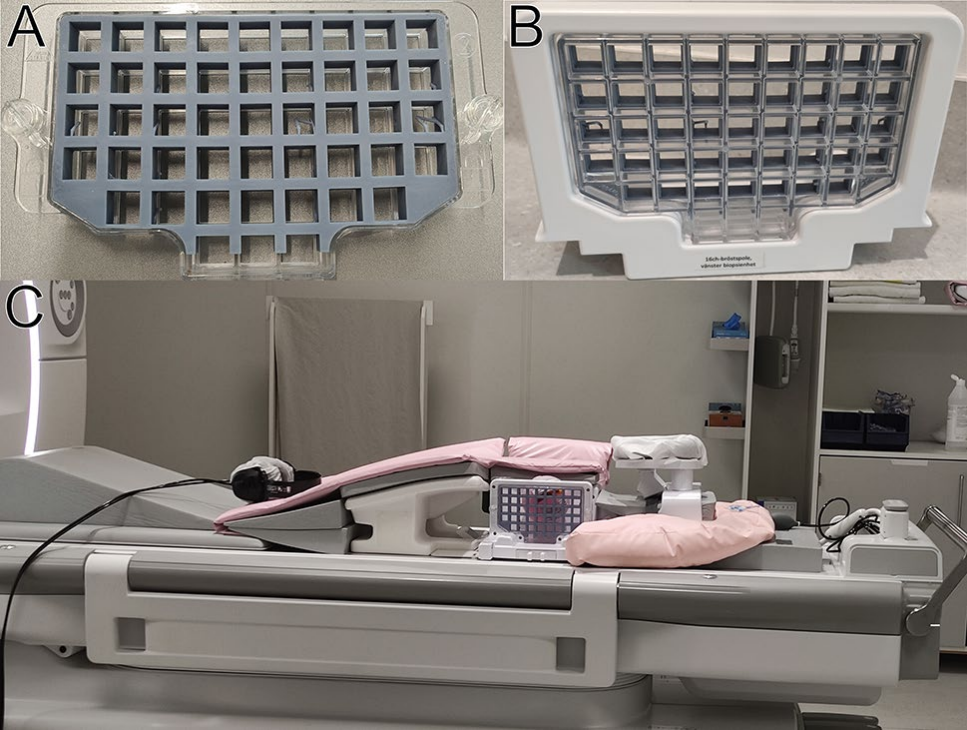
A: The finished part inserts into existing MRI-guided biopsy grid. B: the part on the grid in its retainer. C: The entire MRI-guided biopsy setup on the scanner bed
